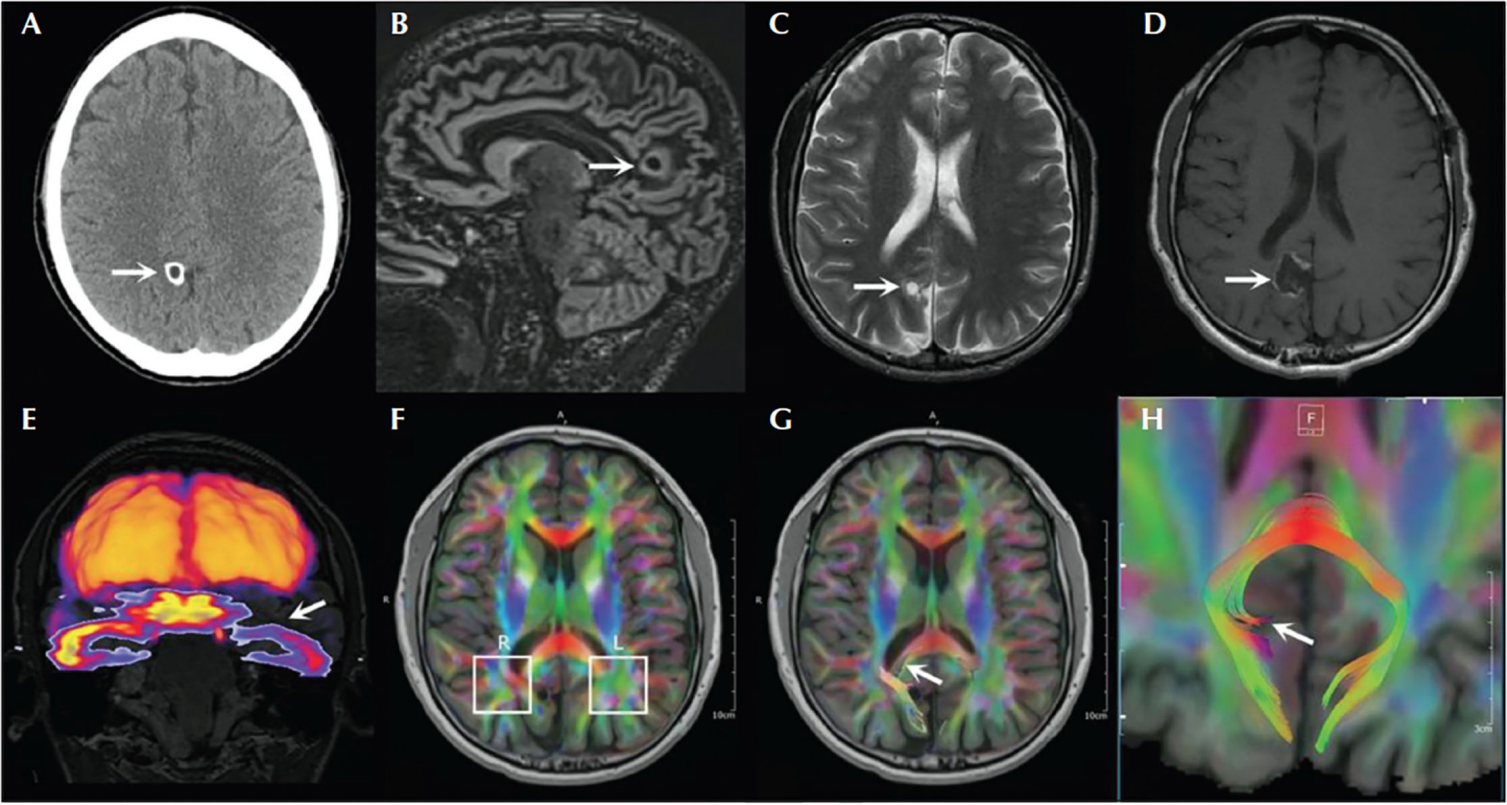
Brain imaging of the patient with right precuneus neurocysticercosis and left temporal lobe seizure involvement. (A) Head CT showing calcified lesion in the right precuneus. (B) Double inversion recovery MRI showing the lesion with surrounding gliosis. (C) T2-weighted image showing the lesion. (D) Postoperative MRI T1 sequence showing the cavity. (E) Coregistration of FDG-PET with MRI showing hypometabolism in the left temporal lobe. (F–H) Diffusion tensor imaging through the level of the lesion showing asymmetrical findings with respect to the parietal white matter [adapted from (8)].
